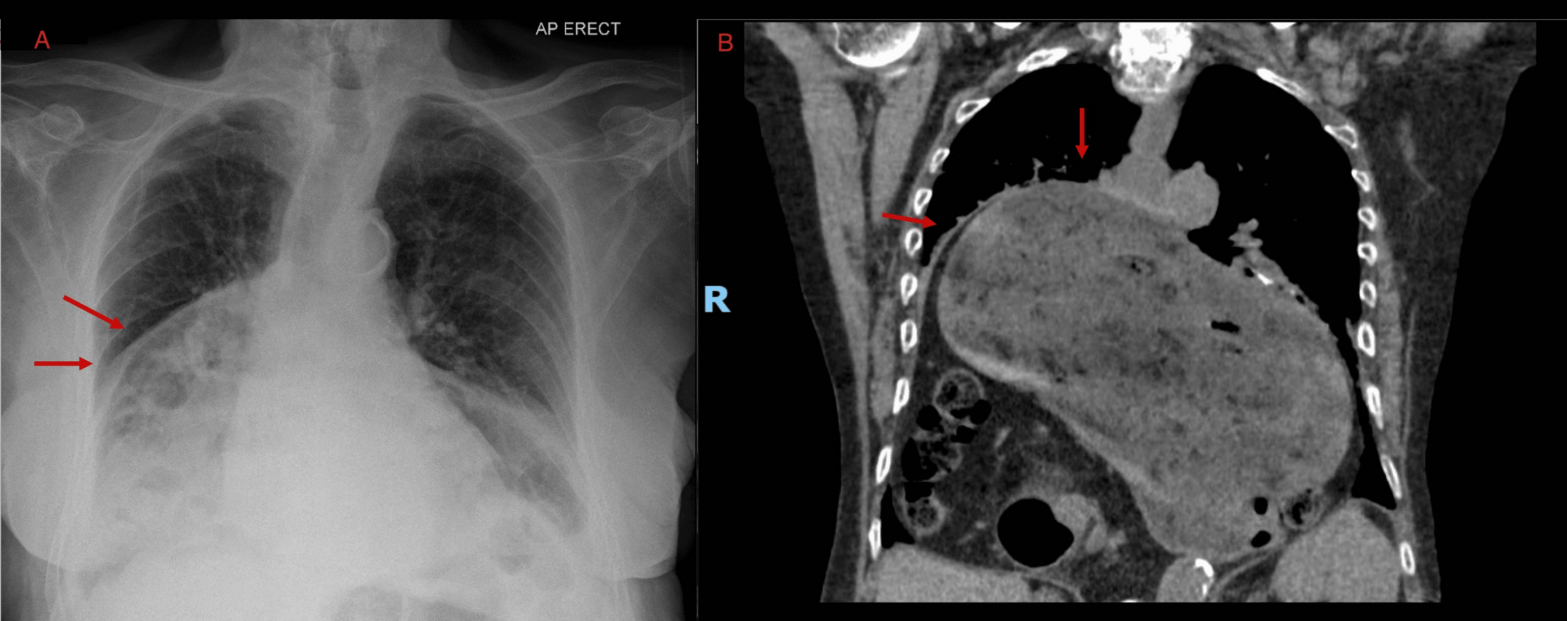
X-ray (A) and CT (B) scan of the chest. (A) Upright chest radiograph demonstrating a large retrocardiac air–fluid level, consistent with a massive hiatal hernia. The cardiac silhouette appears distorted and mildly displaced. (B) Coronal section from CT angiogram of the thorax showing a large hiatal hernia containing the stomach and transverse colon. The herniated viscera are seen exerting significant anterior compression on the heart, displacing it within the thoracic cavity. This mechanical effect mimicked tamponade physiology.
